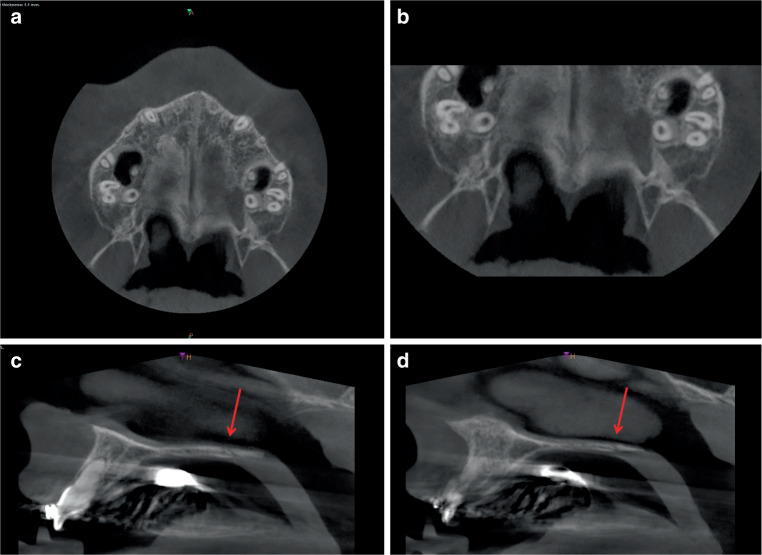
Horizontal (a, b) and sagittal slices (c, d) after combined rapid maxillary expansion/Delaire facemask (RME/DFM) treatment from a cone-beam computed tomography (CBCT) scan of a 12.8-year-old girl. a, b Transverse slice (overview and detail) with open median and open posteriorly curved transverse palatal suture and closed pterygopalatomaxillary sutures. c, d In the sagittal plane, the open transverse palatal suture (red arrows) runs obliquely/angulated on both sides from ventral–cranial to dorsal–caudal Horizontale (a, b) und sagittale Schichten (c, d) nach kombinierter RME/DFM(„rapid maxillary expansion/Delaire facemask“)-Behandlung aus der DVT (digitale Volumentomographie) eines 12,8 Jahre alten Mädchens. a, b Transversale Schicht (Übersicht und Detailausschnitt) mit offener medianer und offener, nach posterior gewölbt verlaufender transversaler Gaumennaht sowie geschlossenen pterygopalatomaxillären Suturen, c, d In der Sagittalebene verläuft die geöffnete Sutura palatina transversa (*rote Pfeile*) auf beiden Seiten schräg/anguliert von ventral-kranial nach dorsal-kaudal
